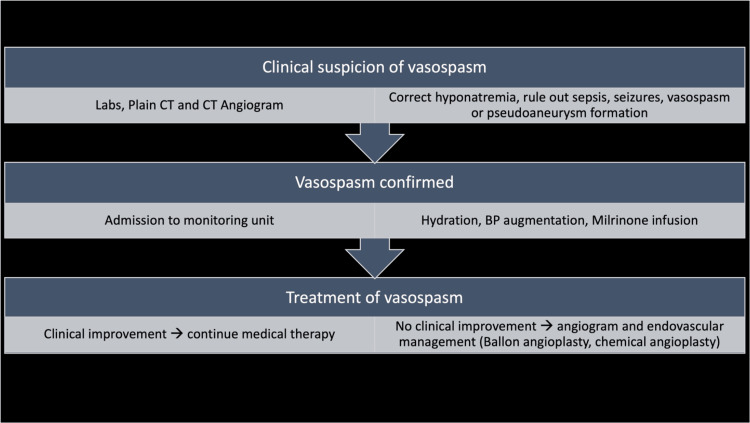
Management algorithm for suspected vasospasm following petroclival meningioma resection CT: computed tomography; BP: blood pressure
